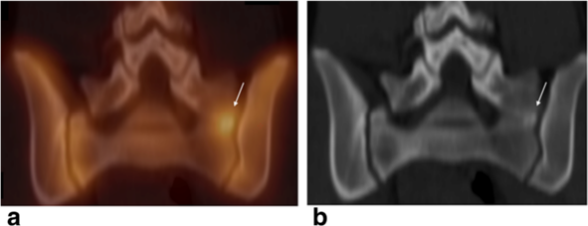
Lumbarization of the S1 vertebra with anomalous articulation of the left side of S1 to the remainder of the sacrum and with the left iliac bone in a 16-year-old female gymnast with left-sided low back pain. a Coronal fused SPECT/CT image shows intense uptake at the left S1–S2 pseudoarthrosis. b Coronal CT image shows lumbarization of S1 with articulation with S2 instead of fusion, with marginal sclerosis seen on the *left side* (*arrow*) likely due to degenerative changes
